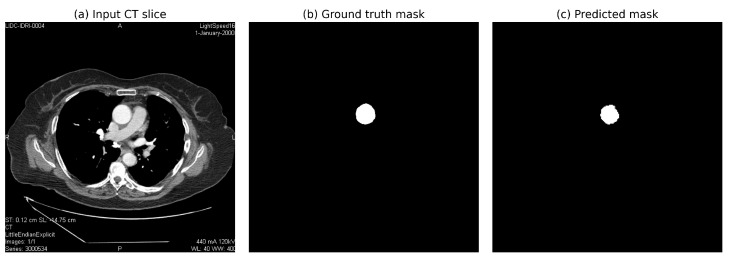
Sample segmented images of the ascending aorta: (a) input CT slice; (b) true mask, (c) predicted mask generated by the U-Net model.
